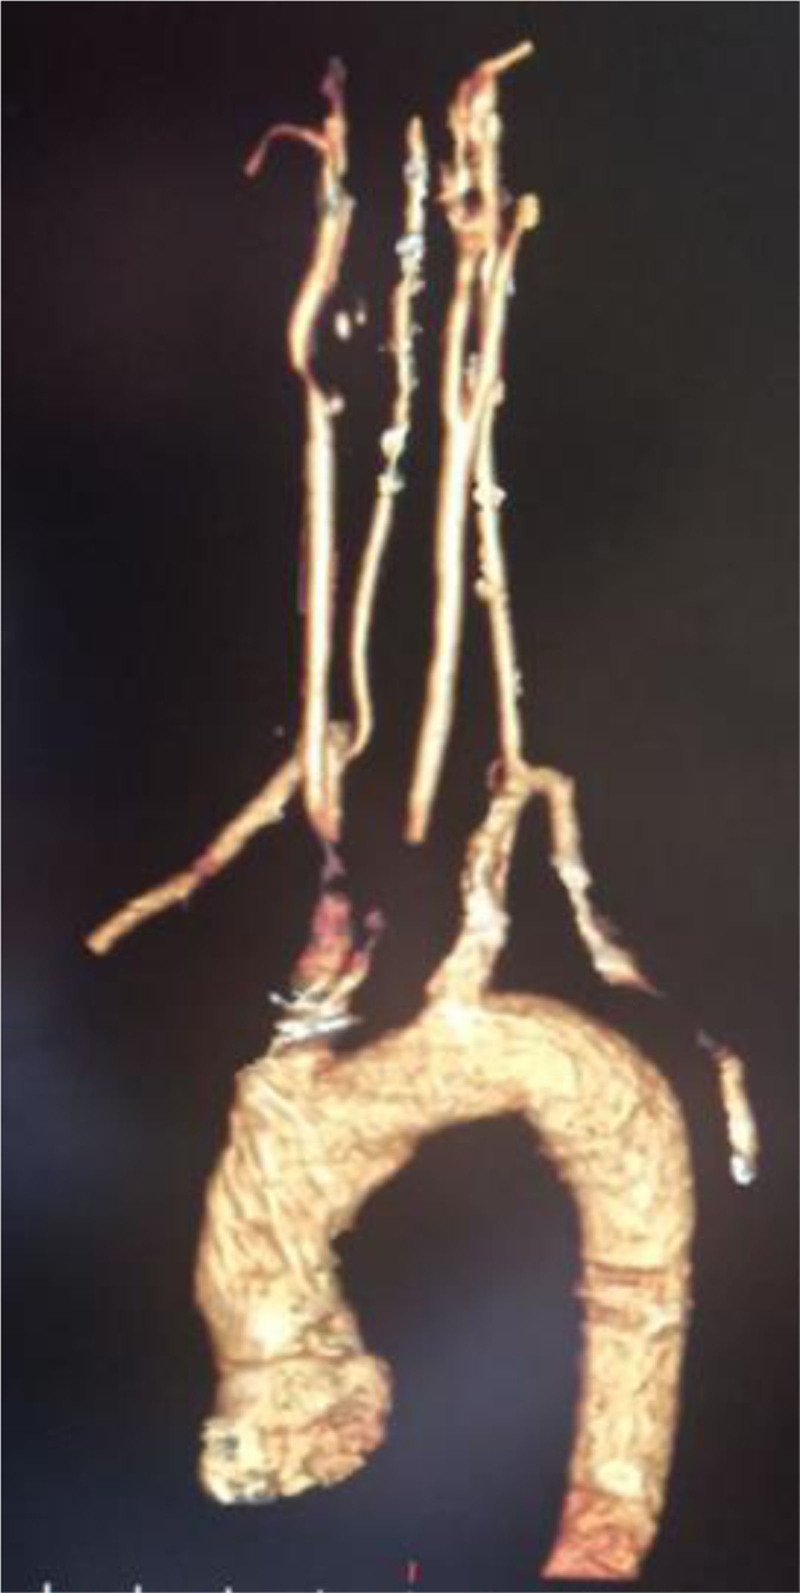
A multisegment CT scan of the aortic arch, its branches, and the carotid arteries showed that the left common carotid artery had a common origin with the brachiocephalic trunk, with acceptable continuity at a distance of 27 mm, followed by an interruption at a distance of 16 mm, and then good recurrence. The right subclavian artery exhibited vascular interruptions 2 cm after its origin spanning 15 mm. The right common carotid artery showed an annular interruption at its beginning followed by recurrence. The right external carotid artery demonstrated 50% annular stenosis at its origin over 5 mm. The right internal carotid artery appeared to be interrupted for 18 mm before recanalization. CT = computed tomography.
